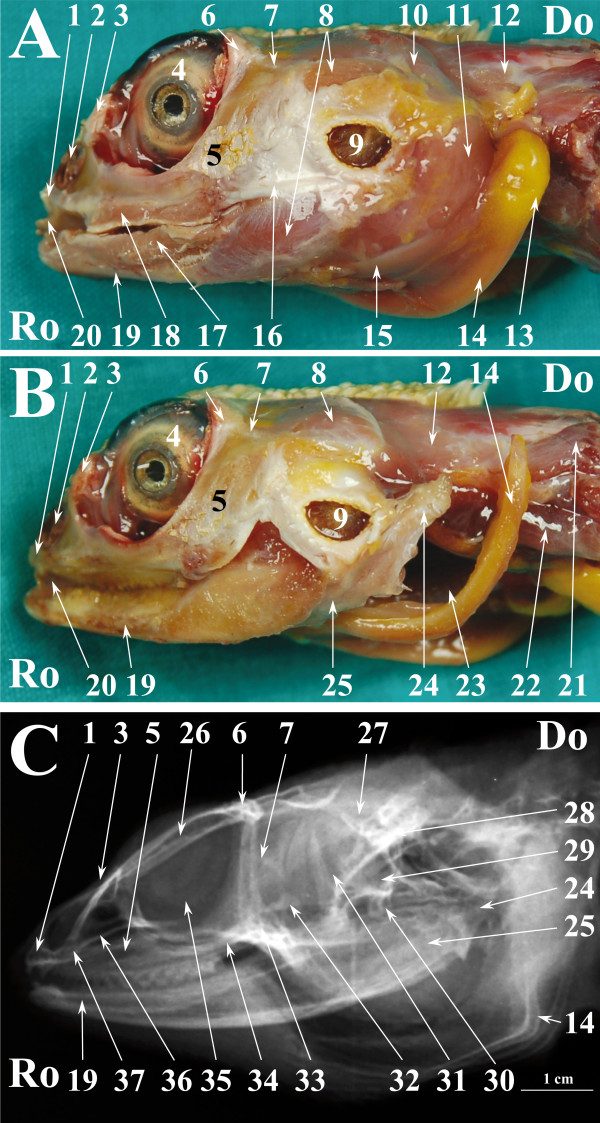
Normal radiographic anatomy of the head of the bearded dragon in LL projection.A. LL photographic image in a superficial plane of the stratigraphic dissection of the head of a bearded dragon (only skin was removed). B. LL photographic image in a deep plane of the stratigraphic dissection of the head of a bearded dragon. C. LL radiographic projection of the head of a bearded dragon. Ro is rostral, Do is dorsal. 1. Premaxillary bone; 2. Nostril; 3. Prefrontal bone; 4. Eye; 5. Maxillary bone; 6. Postorbital bone; 7. Jugal bone; 8. Musculus adductor mandibulae externus superficialis; 9. Ear; 10. Musculus adductor mandibulae externus medialis; 11. Musculus pterygoideus typicus; 12. Musculus depressor mandibulae; 13. Fat body; 14. Ceratobranchial process of hyobranchial skeleton; 15. Musculus sphincter colli; 16. Quadrato maxillary ligament; 17. Infralabial glands; 18. Supralabial gland; 19. Dentary bone; 20. Tongue; 21. Musculus trapezius + Musculus clavicle dorsalis; 22. Musculus episternocleidomastoid; 23. Trachea; 24. Articular bone; 25. Angular bone + Surangular bone; 26. Frontal bone; 27. Parietal bone; 28. Squamosal bone; 29. Paraoccipital process of otooccipital bone; 30. Quadrate bone; 31. Epipterygoid bone; 32. Coronoid bone; 33. Ectopterygoid bone; 34. Pterygoid bone; 35. Scleral ossicles; 36. Palatine bone; 37. Vomer bone.
